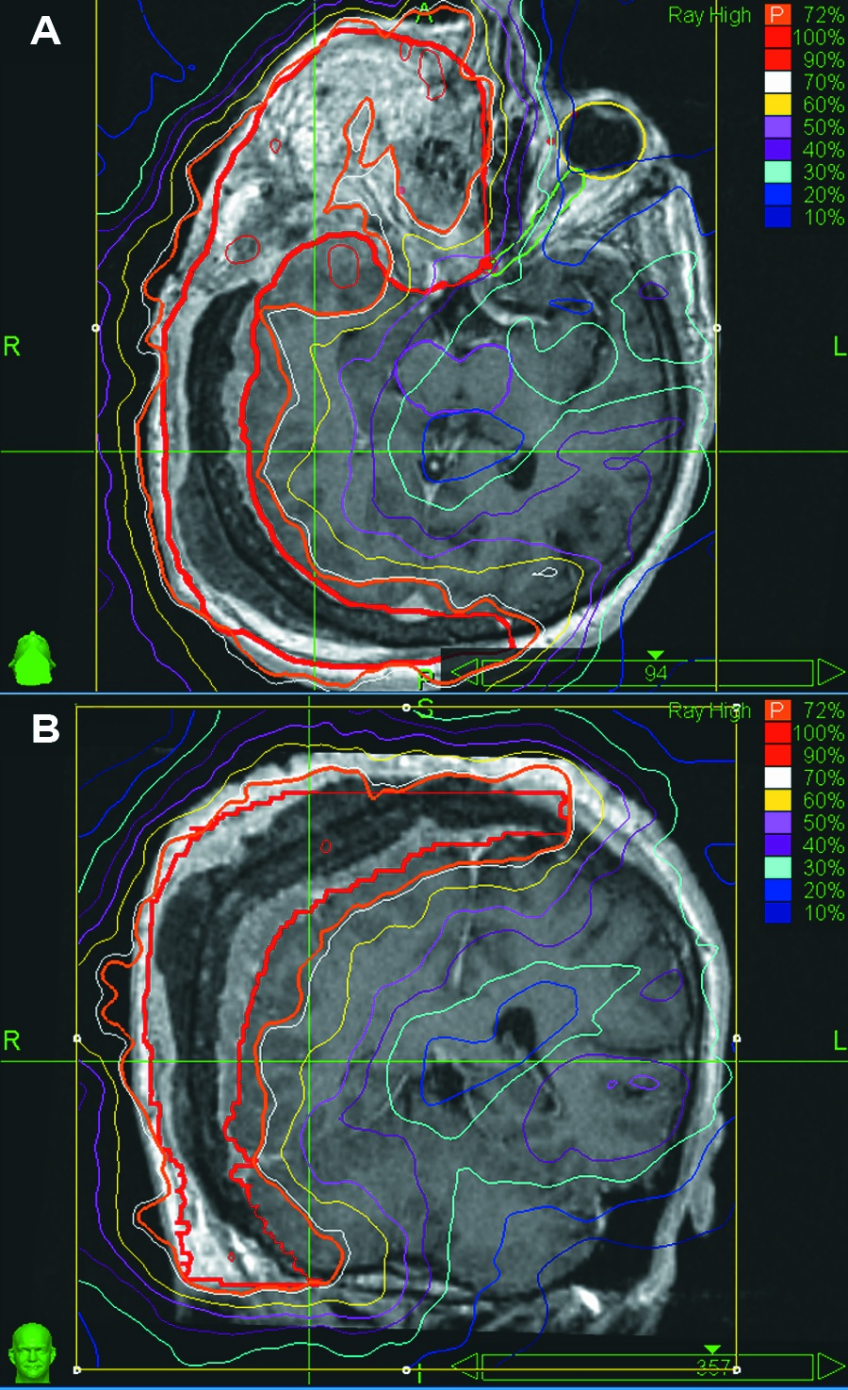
Tumor extent with MRI and isodose distributions A - axial plane, B - coronal plane
